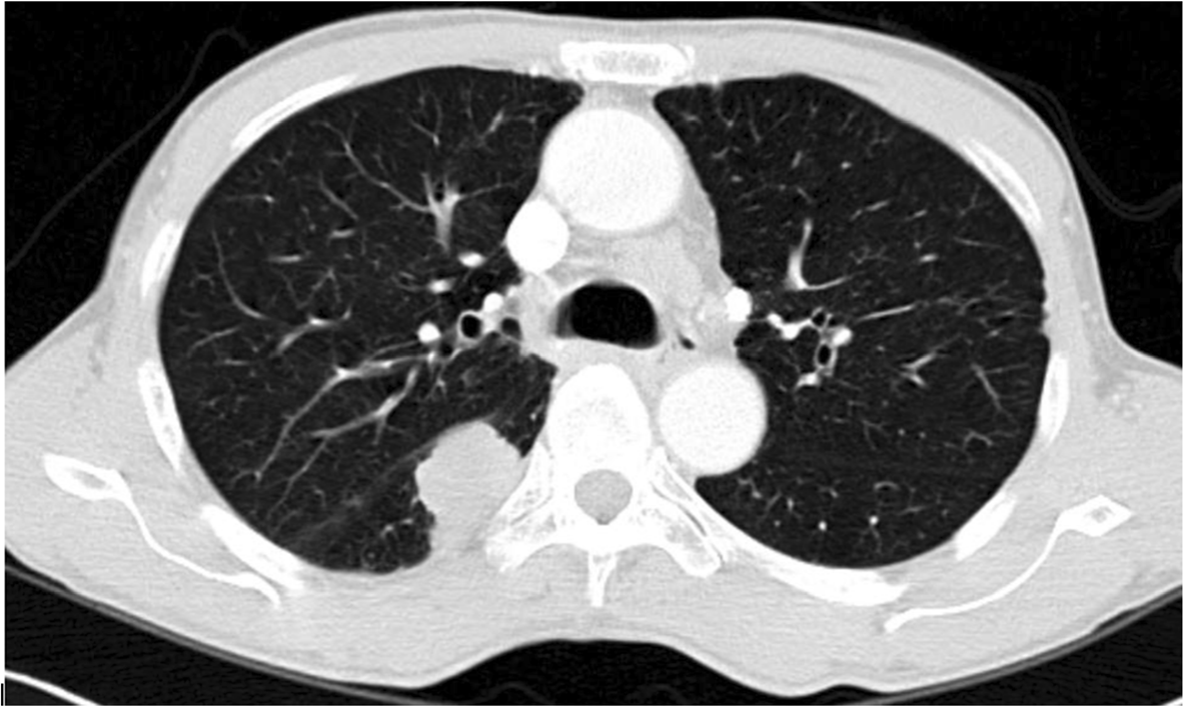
A contrast-enhanced chest CT shows a nodular mass in the superior segment of the right lower lobe.
